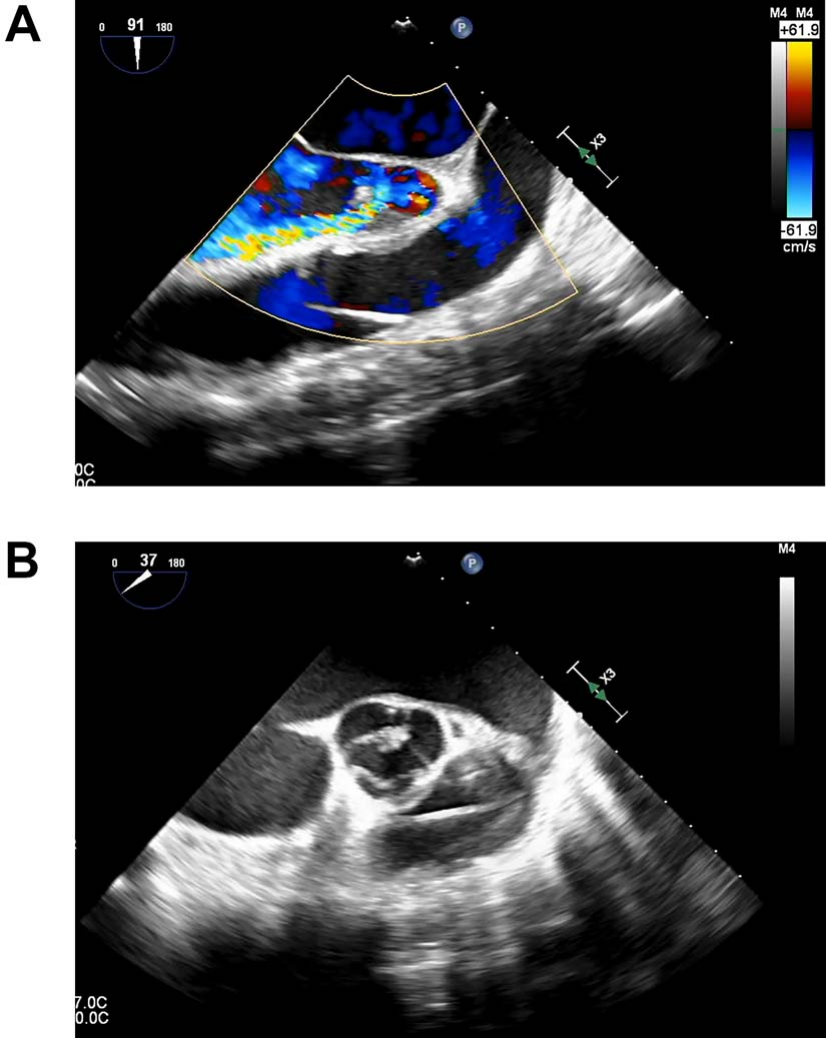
(A). Transesophageal echocardiography showing severe AI at 1 day after Heart Mate III implantation. (B). Transesophageal echocardiography showing the coaptation stitch in the right and noncoronary cusps had failed at 1 day after Heart Mate III implantation.
